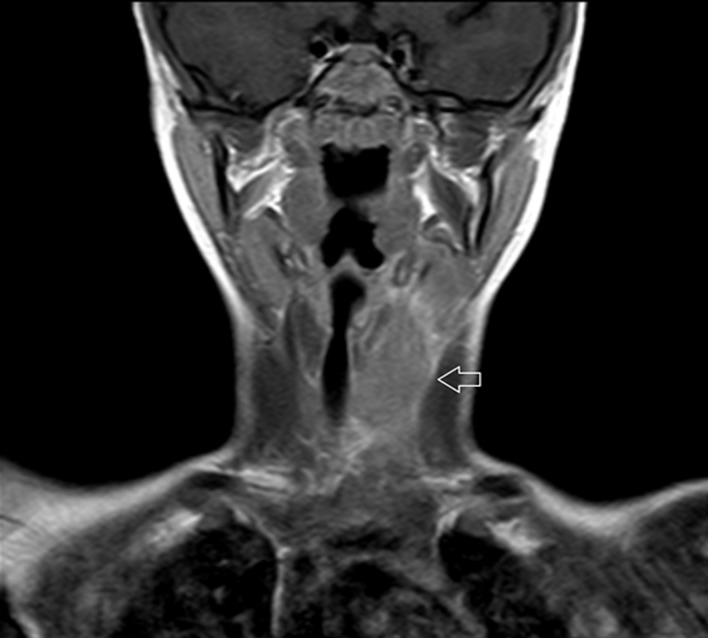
coronal T1-w MRI, enlargement of the left thyroid lobe which has lost normal signal intensity, note obliteration of interposed fat planes with sternocleidomastoid muscle, mild right trachea deviation is obvious, normal flow void is noticed from patent left main neck vessels
